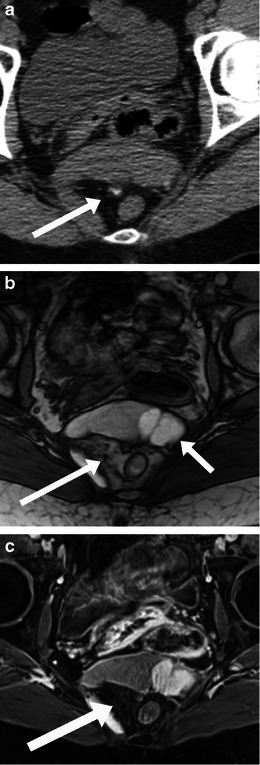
Imaging of sacrococcygeal teratoma. A 49-year-old woman with benign cystic teratoma. a Unenhanced axial CT scan performed for renal colic showed a heterogeneous presacral mass with areas of soft-tissue density, fat density and a speck of calcification (*arrow*). Subsequent MRI shows areas of fat which show signal drop (*long arrows*) on the T1-weighted fat saturated images (c), and areas of haemorrhage (*short arrows*, b) which do not show signal drop
